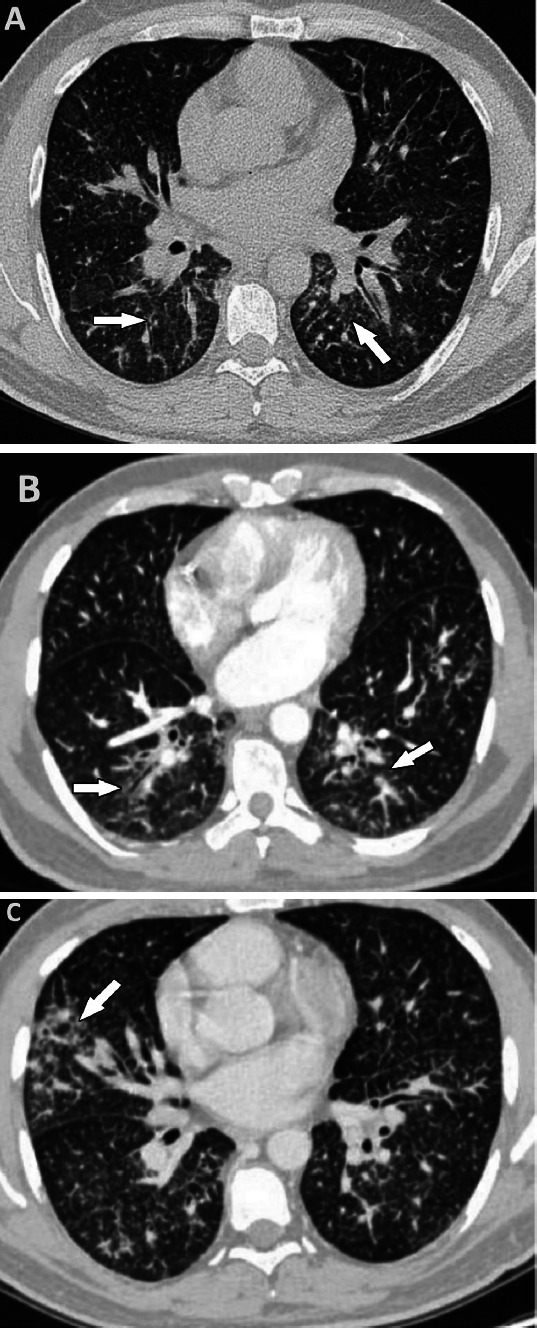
Three High resolution computed tomography [HRCT] scan carried out one month apart showing progressive Ground Glass Opacities and nodular septal thickening. A) First HRCT. B) Second HRCT. and C) Third HRCT.
